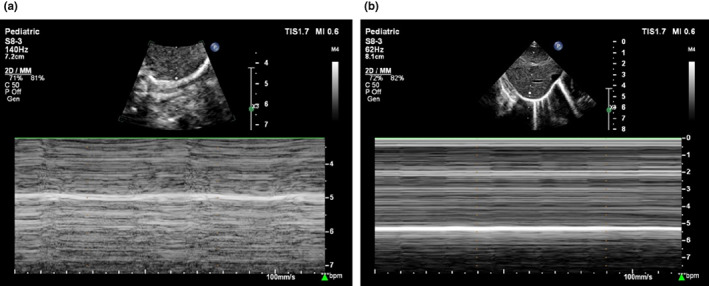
Diaphragm ultrasound: Deduced bilateral diaphragmatic activity, (a) Left side, (b) Right side
